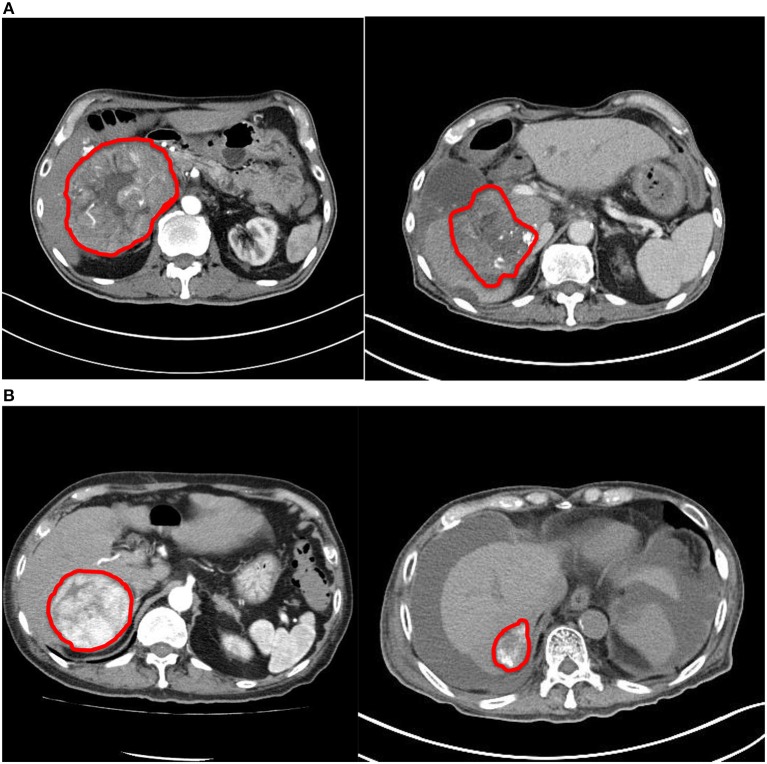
Comparison of computed tomography images before and after treatment. (A) Patient #2. (B) Patient #4. Both tumors demonstrated significant reduction in size and enhancing component after treatment.
